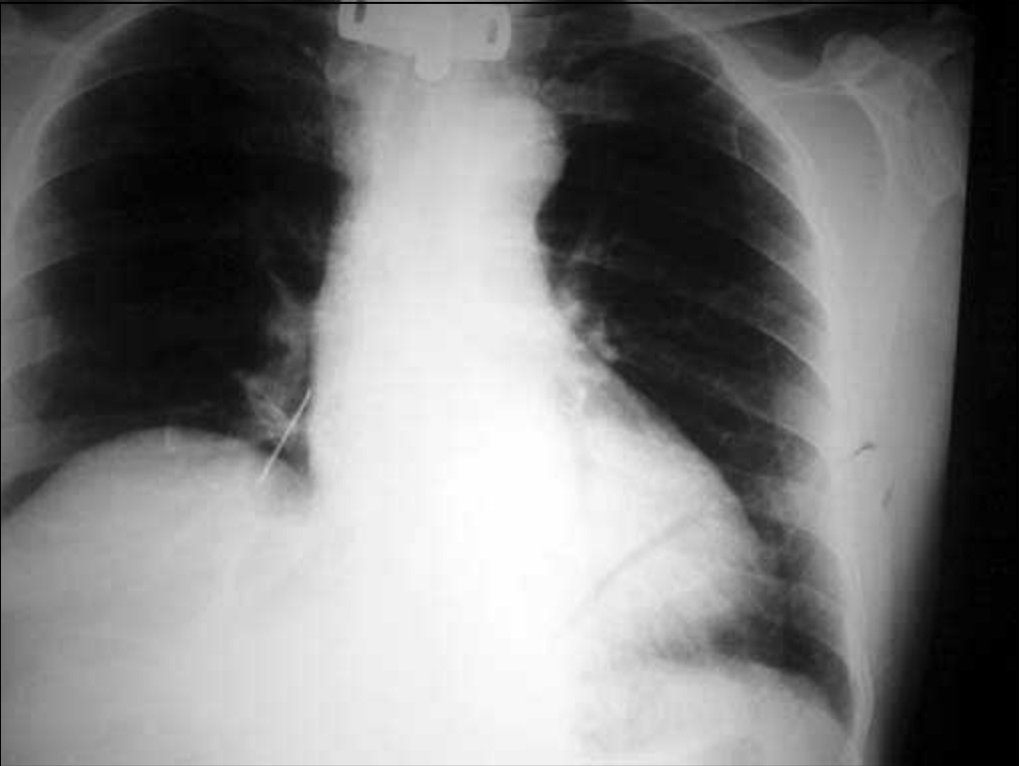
Chest X-ray in PA showing foreign body in right source bronchus.
